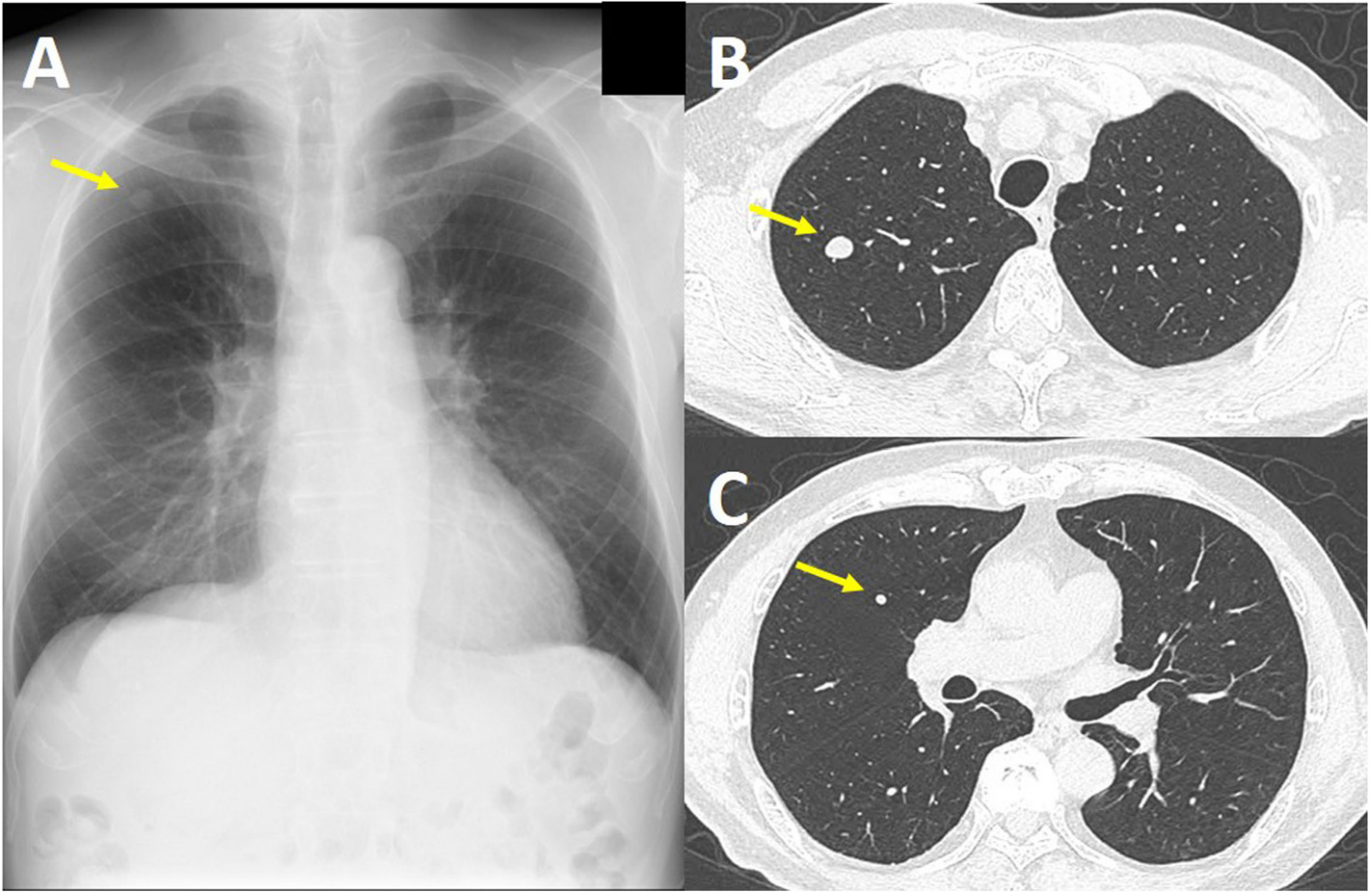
Imaging of the recurrent lesions in the lung. a Chest X-ray demonstrated a well-defined nodule in the right upper lung field (*arrow*). b, c Chest CT demonstrated two round nodules in segments 1 and 3 of the right lung, respectively (*arrow*)
